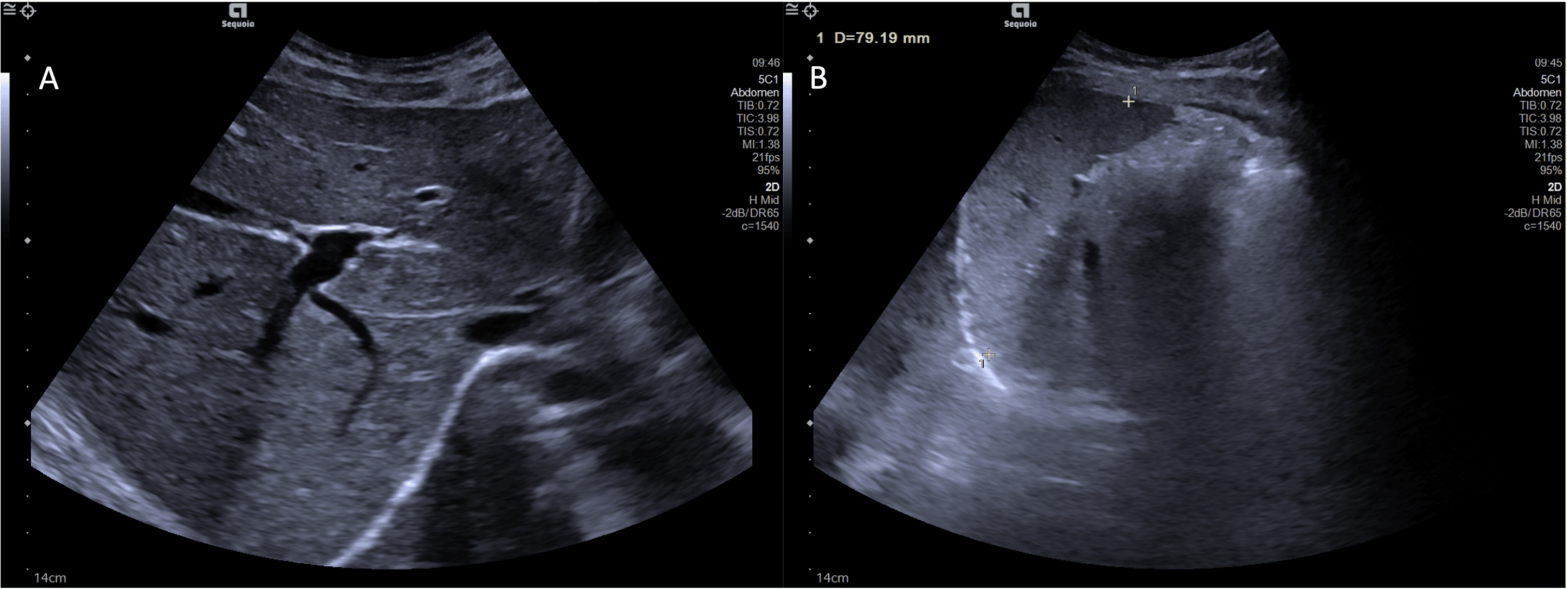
Abdominal ultrasound A) Liver of normal size, with regular contours and homogeneous echotexture. Bile ducts are not dilated, and the portal vein and hepatic veins are of normal caliber.
